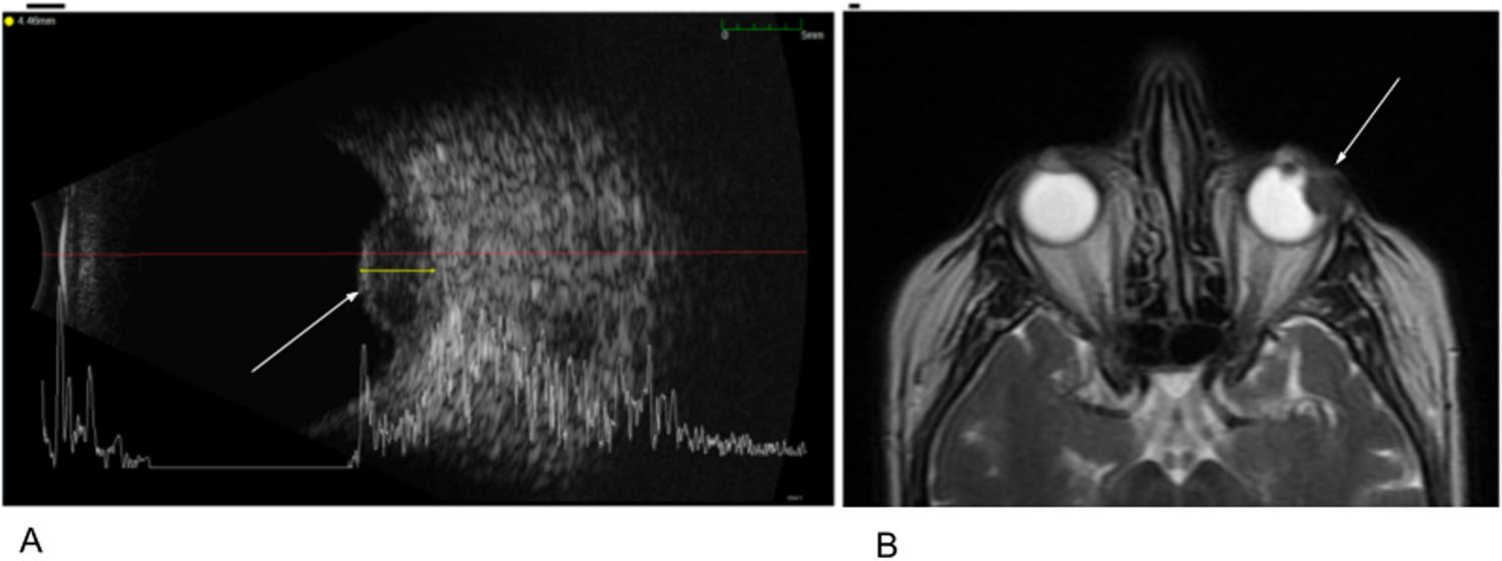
A Left eye, ophthalmic ultrasound of choroidal melanoma (arrow). Note the elevated nature of the lesion in the retina projecting into the vitreous, the gel-like fluid that fills the eye. B Left eye, T2-weighted MRI of choroidal melanoma (arrow). Note the low signal intensity lesion and elevation
